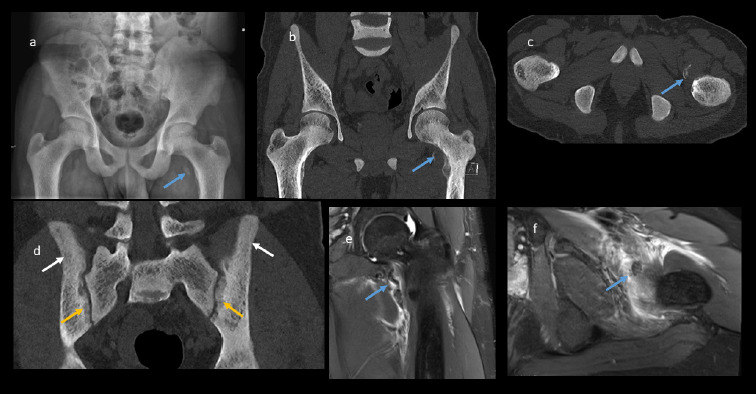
a) plain radiograph of pelvis, b) coronal c) axial CT reformats of pelvis showing avulsion fracture of the left lesser trochanter (blue arrow) and d) coronal reformats of both SI joints showing diffuse bony sclerosis (white arrow) and sub periosteal bone resorption (orange arrow) of both SI joint. e, f) Coronal and axial PD FAT SAT MRI showing avulsion fracture (blue arrow) of left lesser trochanter (psoas attachment site) with associated extensive soft tissue swelling and oedema.
